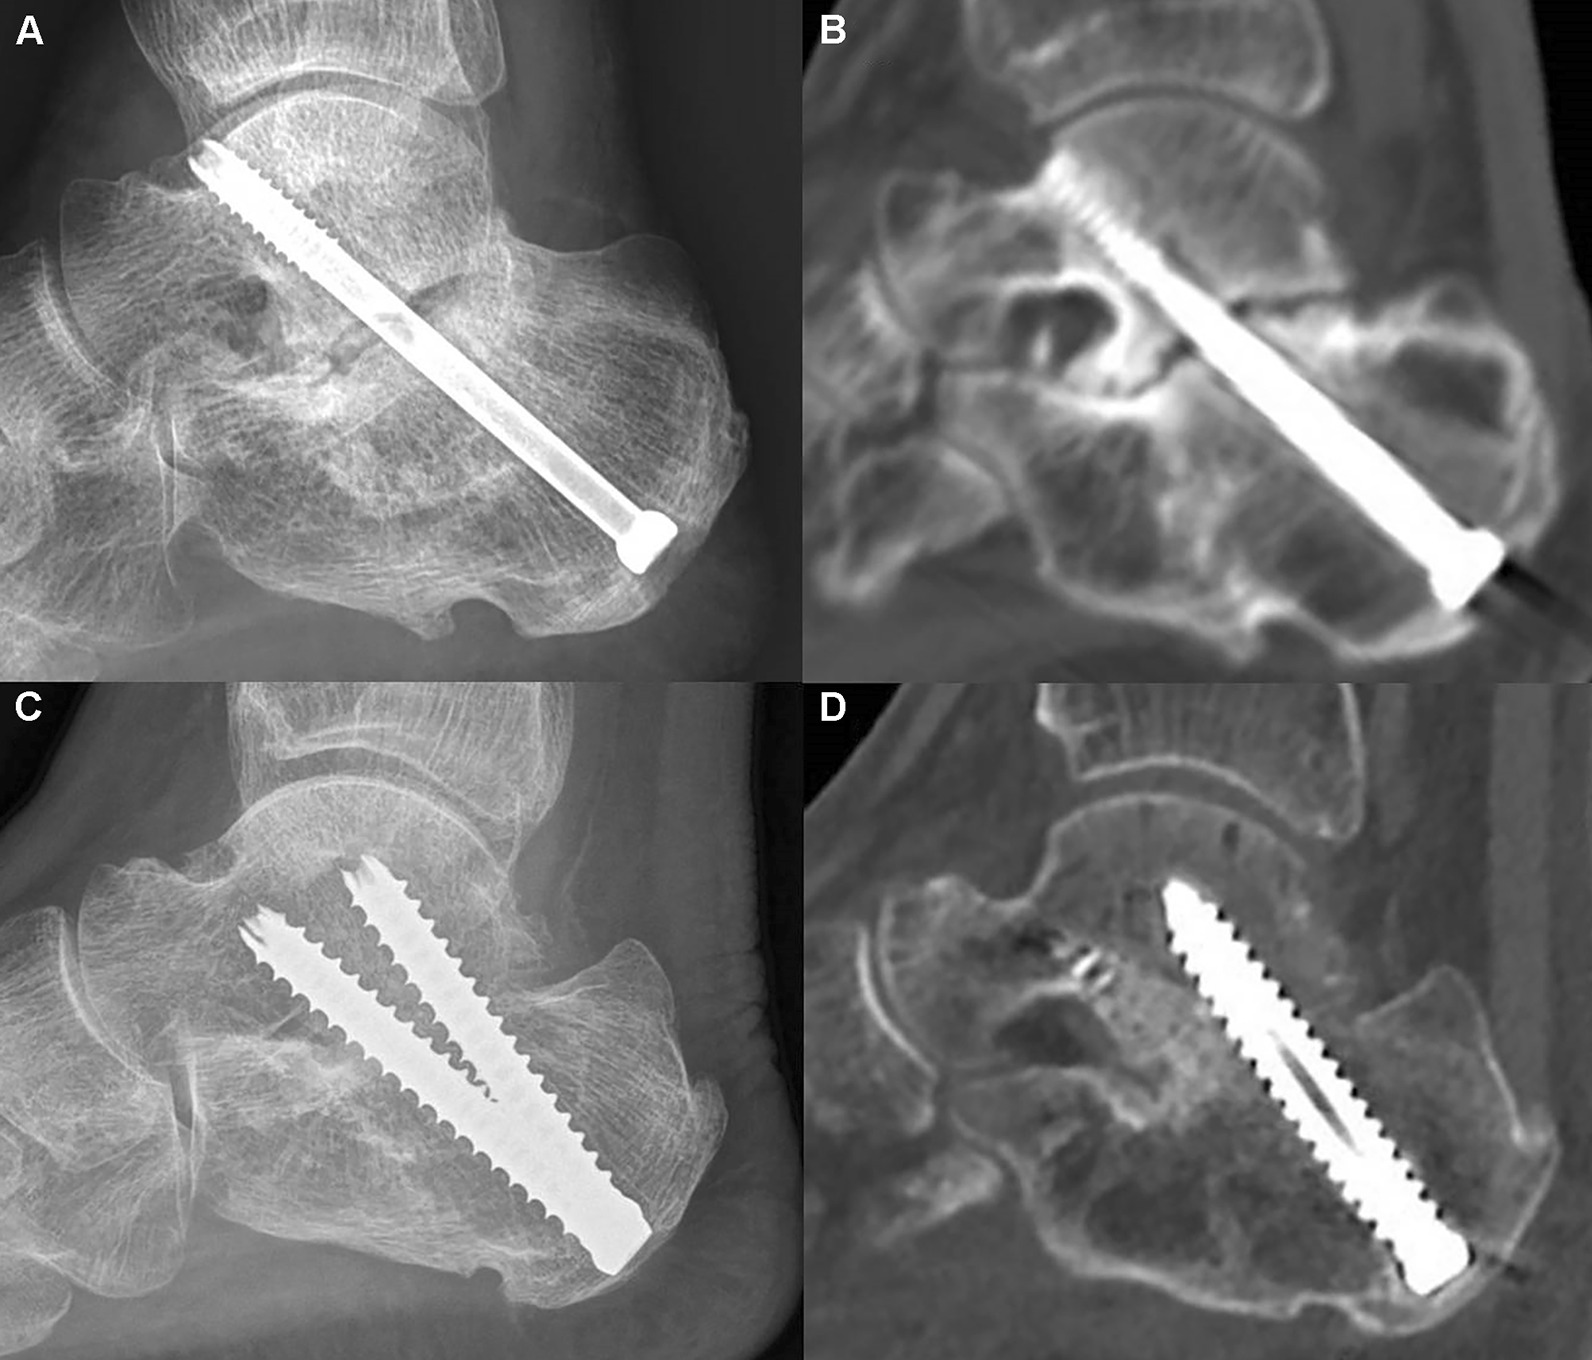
a, b Nonunion was observed on the lateral radiograph and CT scan of a 67-year-old male patient after using a single partially threaded screws for the subtalar arthrodesis. c, d Bone union was observed in a 56-year-old male patient after using two fully threaded screws in divergent configuration
